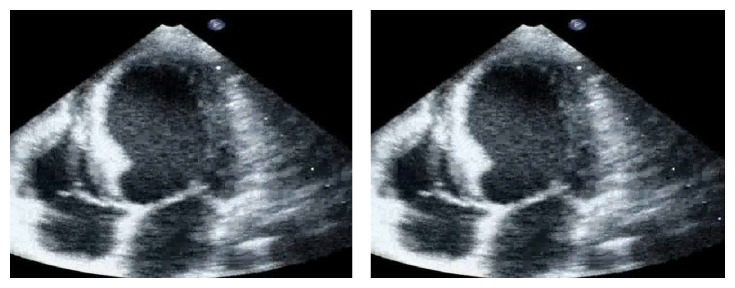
Transthoracic echocardiogram performed in the ED showed akinesia of left ventricular medium-distal apical septum and medium-distal anterior walls, with a 40% EF (see video).
